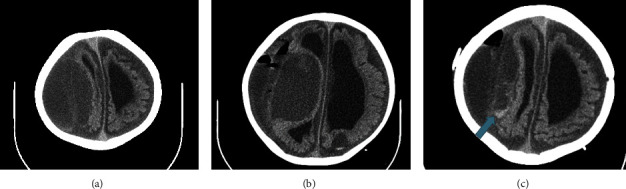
Nonenhanced axial CT scan demonstrates large right temporoparietal fluid collection (a). Nonenhanced axial postop CT scan demonstrates failure of surgical drainage due to the presence of dural layer in deep portion of the collection (b). Follow-up nonenhanced axial CT scan showed interval hemorrhage (blue arrow) in the collection (c).
